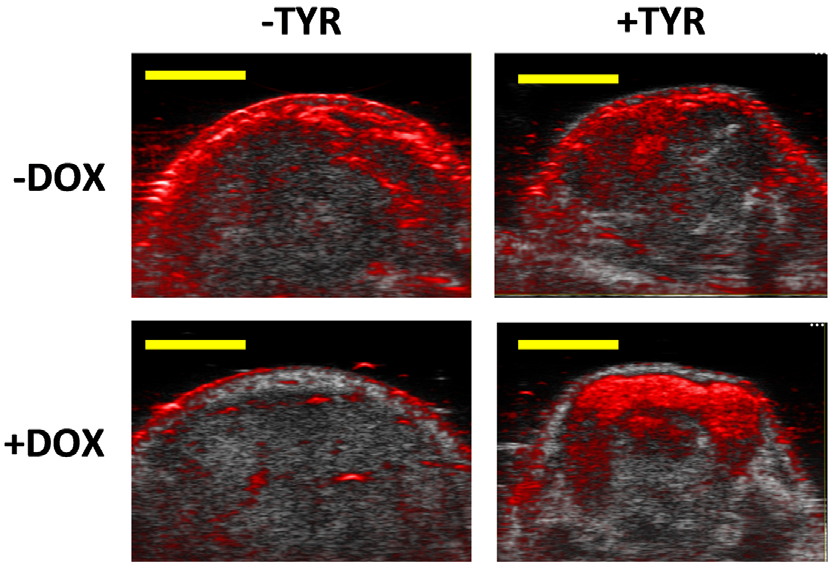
Photoacoustic (680 nm) and micro-ultrasound (grayscale) co-registered imaging of -TYR and +TYR tumors with and without DOX treatment. Scale bars represent 2 mm.
